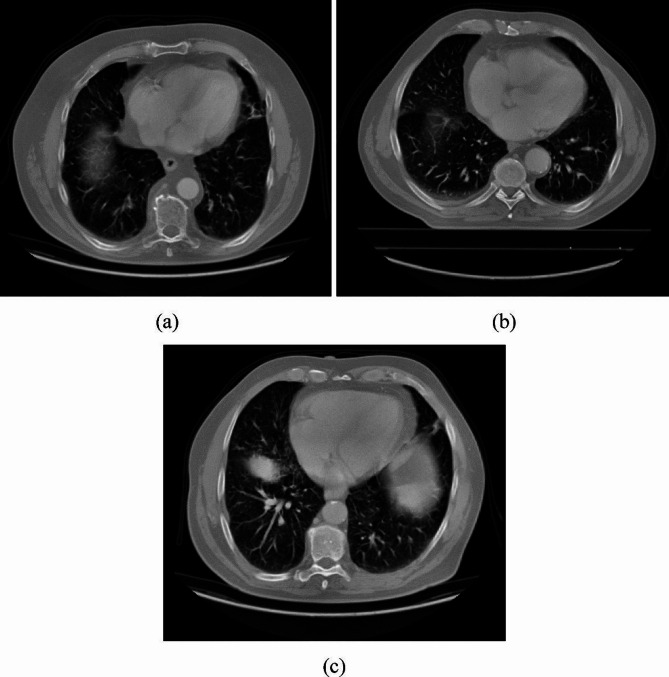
O/Ps of WOA-PIQE-CLAHE framework on representative CT images (a) CT slice 1 (b) CT slice 2 (c) CT slice 3.
